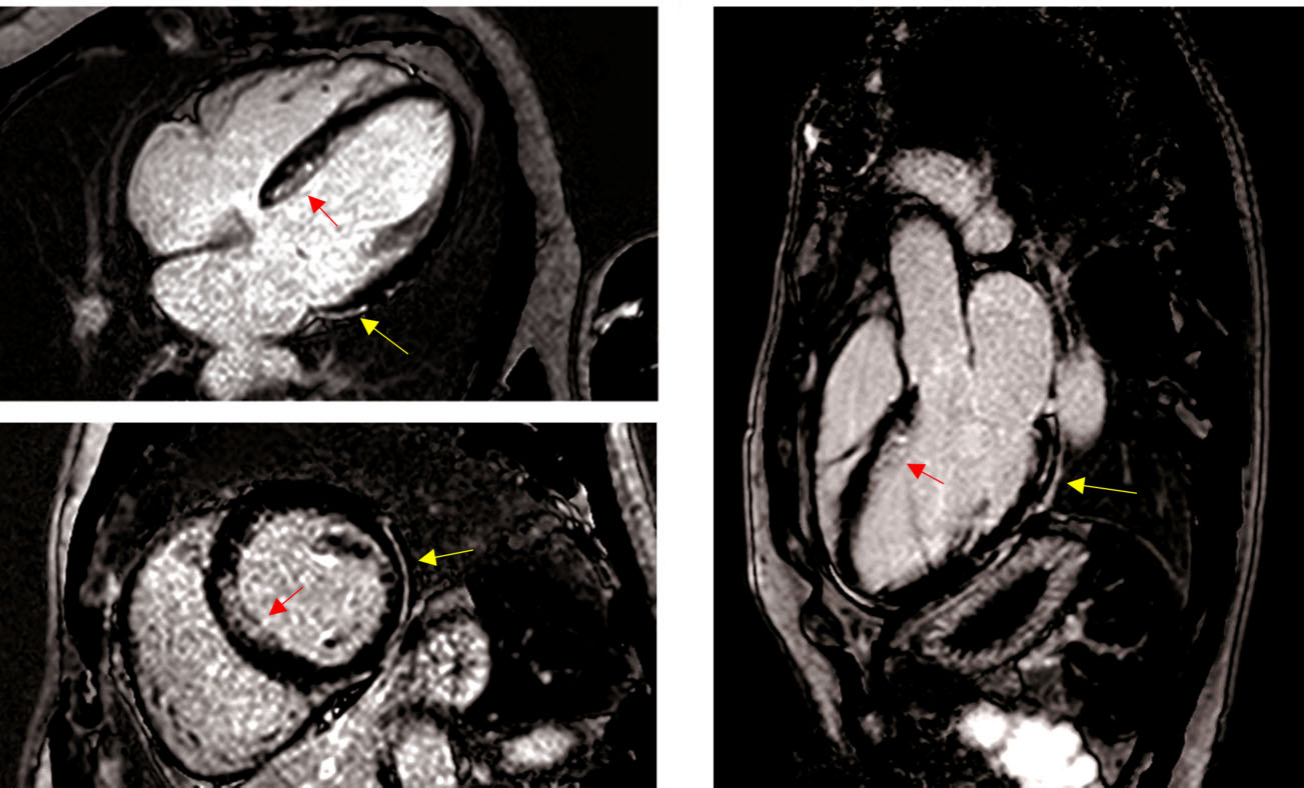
Late gadolinium enhancement cardiovascular magnetic resonance images showing horizontal long axis (four-chamber) (*A*) mid-ventricular short axis (*B*) and left ventricular outflow tract (three-chamber) (*C*) views. The yellow arrows indicate regions of epicardial lateral fibrosis and the red arrows point to septal subendocardial enhancement.
